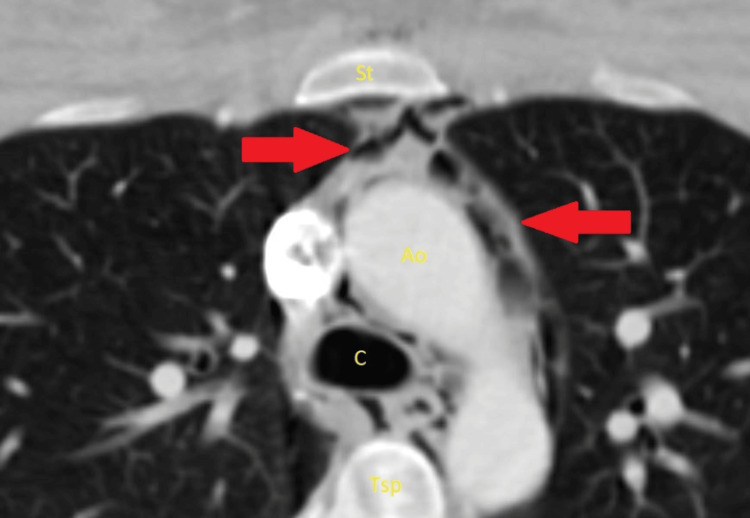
An axial CT image through the upper mediastinum is shown. The arrows indicate foci of gas within the mediastinum, located in retrosternal space and extending around the aorta and carina. St, sternum; Ao, aorta; C, carina; Tsp, thoracic spine.
